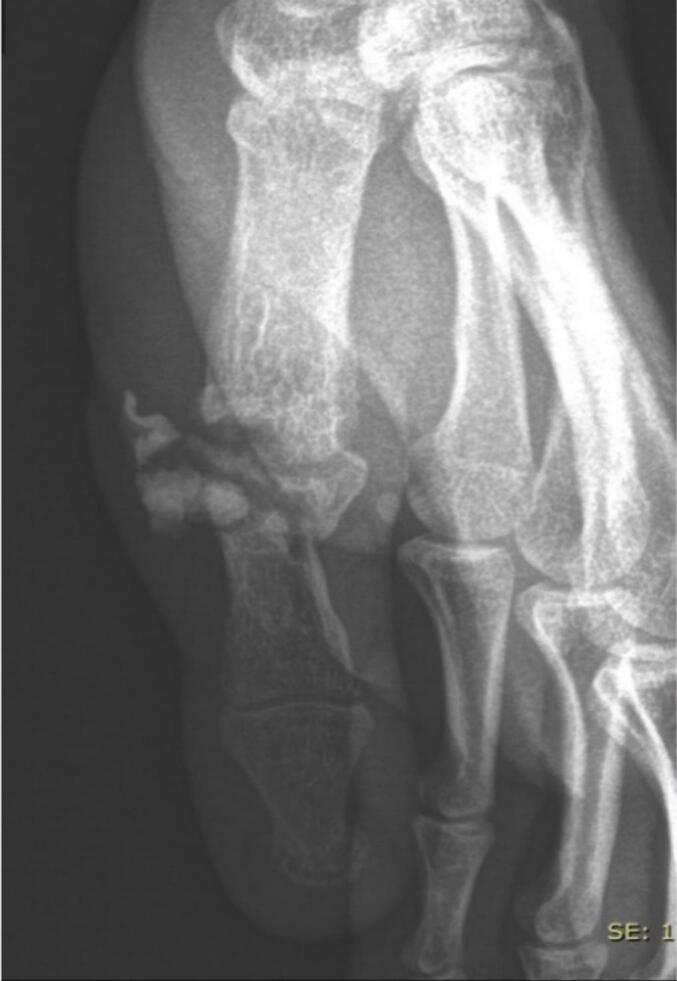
Frontal radiograph at 3 weeks showing osteoarthritis, necessitating the removal of the osteosynthesis material.
